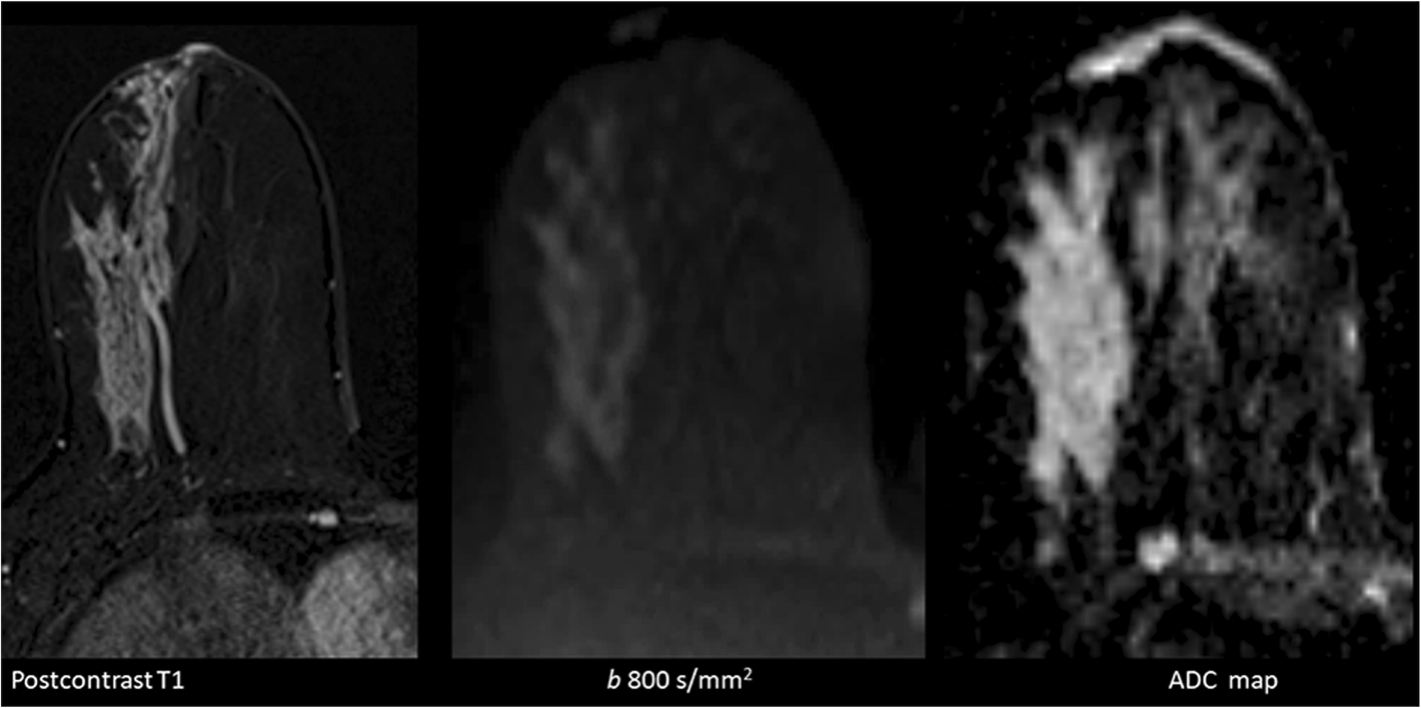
Segmental enhancement and corresponding increased diffusion due to DCIS. The interpretation of DCIS at DW-MRI remains controversial. Depending on the cellularity, diffusion features will change
